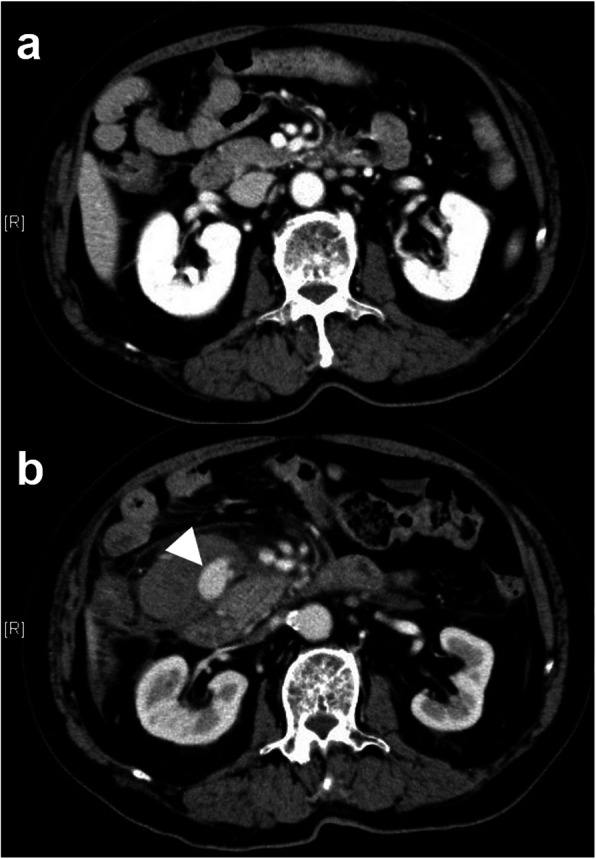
Contrast-enhanced computed tomography (CT) findings at the previous hospital. a CT examination at admission to the previous hospital showed no abnormal findings around the superior mesenteric artery and vein or the duodenum. b CT images obtained on the fourth day after symptom onset demonstrated a newly developed hematoma with a pseudoaneurysm (white arrowhead) in the mesentery of the duodenum
